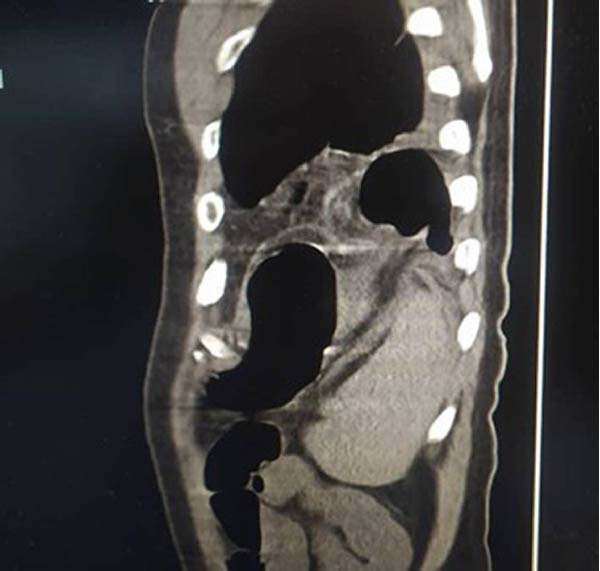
A sagittal CT image showing the incarceration of the splenic flexure with omentum from the abdomen within a diaphragmatic hernia.
